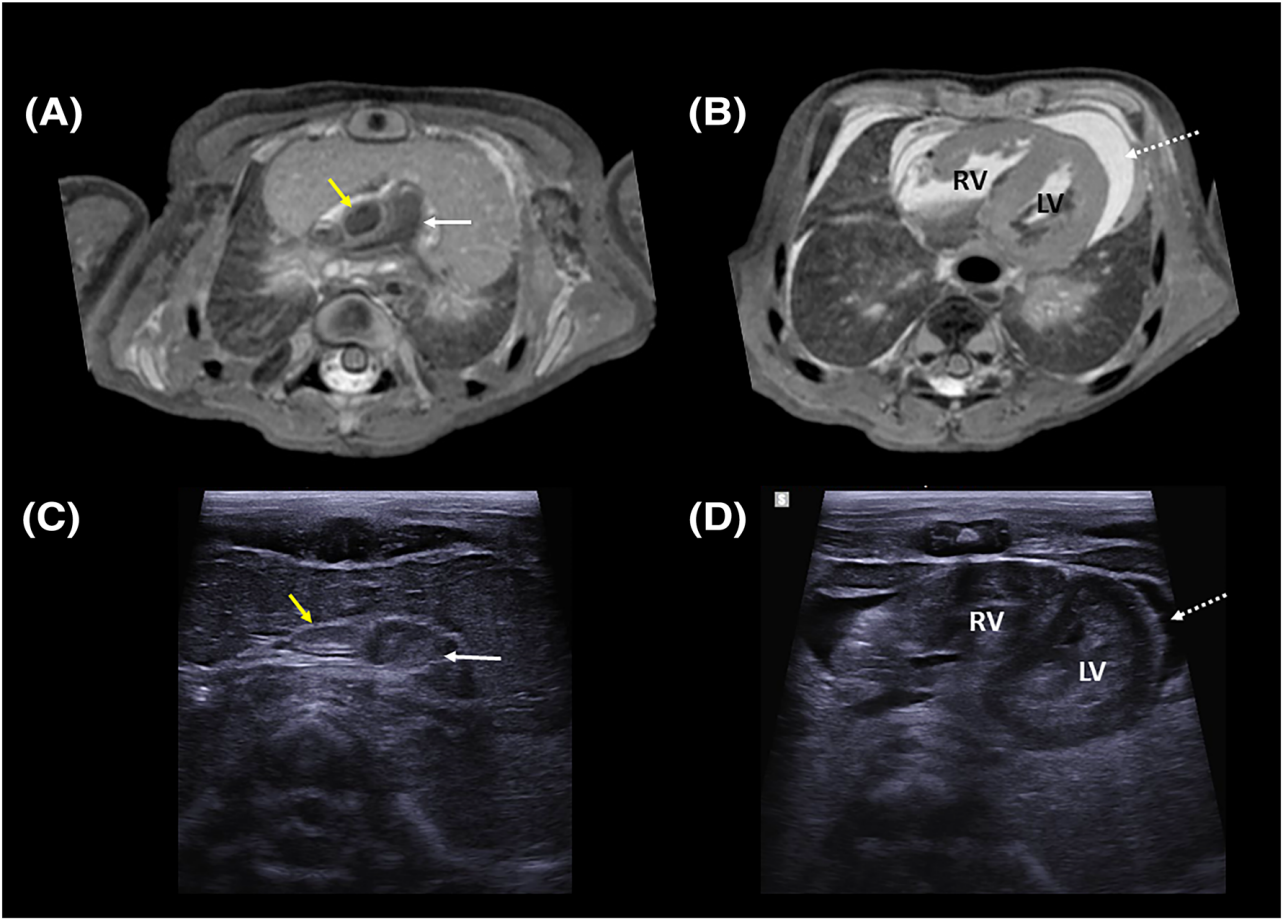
An example of diagnostic quality post‐mortem ultrasound images in a 37‐week gestational aged fetus. The axial T2 weighted post‐mortem MRI images through A, the cardiac outflow tracts and B, the long axis of the heart are compared with transverse ultrasound images in C, and D, These show how several anatomical structures including the main pulmonary artery (white arrow), ascending aorta (yellow arrow), right ventricle (RV), and left ventricle (LV) are visualised in both modalities. A large pericardial effusion (dashed arrow) is also visualised on both MRI and ultrasound [Colour figure can be viewed at http://wileyonlinelibrary.com]
